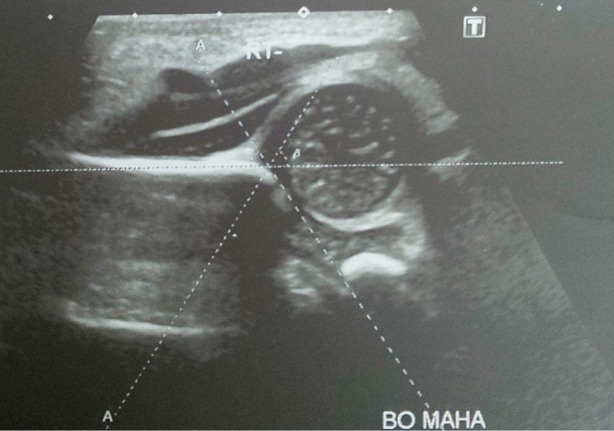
The alpha and beta angles measured on Graf’s Method Ultrasound.
